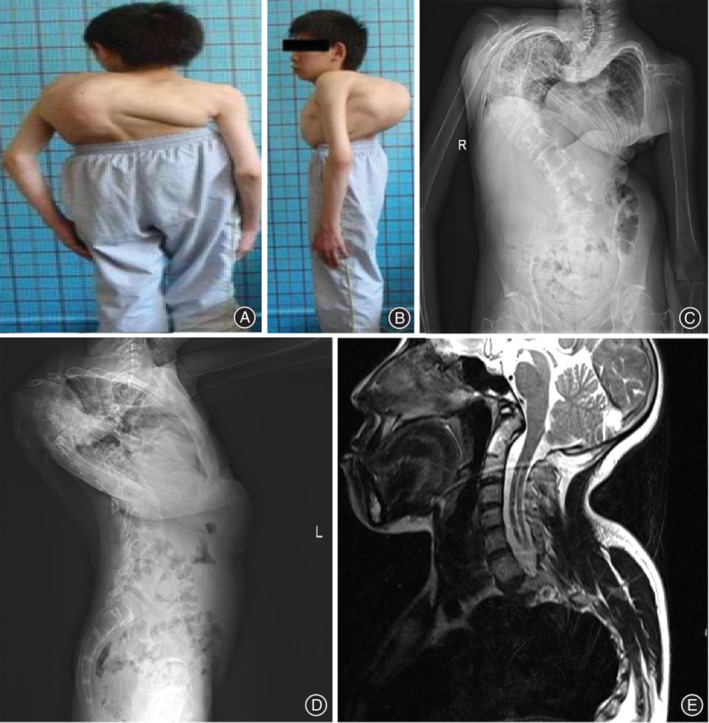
A 17‐year‐old boy with severe kyphoscoliosis associated with cervical osseous abnormalities (COA) and cervical intraspinal neural axis abnormalities (CIINAA). The preoperative appearance reveals spinal deformity (A, B). The preoperative AP and lateral radiographs show the severe kyphoscoliosis (C, D). The sagittal MRI scanning of the cervical spine shows the C_2_–C_3_ fusion, basilar invagination, and Chiari malformation with expanding central canal (E).
